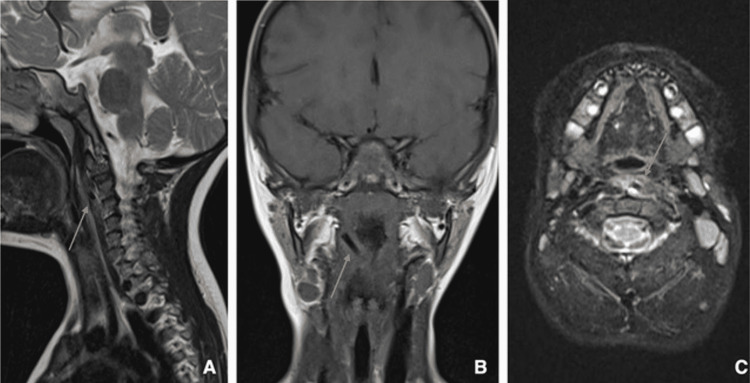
Sagittal T2-weighted (A), coronal T1-weighted (B) and axial T2-weighted with fat saturation (C) planes of the head and neck MRI. (A) Cranial portion of the foreign body (orange arrow) perforating the left longus colli muscle associated with high intensity T2-weighted changes within the muscle; (B) Right extremity of the foreign body (orange arrow); (C) Infiltration and thickening of the left longus colli muscle near to the foreign body (orange arrow).
